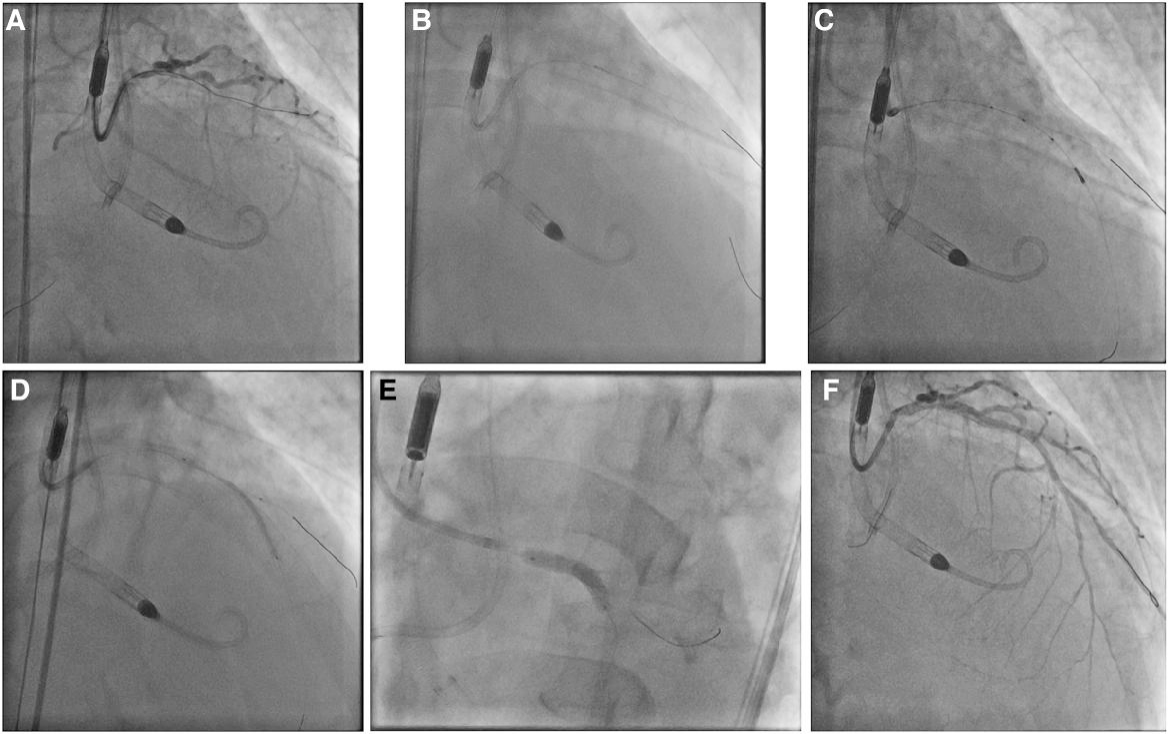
Percutaneous coronary intervention (PCI) of left anterior descending artery (LAD) chronic total occlusion (CTO). (*A*) Gaia Next 1 crossed LAD CTO and passed to 1st diagonal branch. (*B*) Sasuke assisted wiring of distal LAD with Runthrough Floppy wire. (*C*) Intravascular ultrasound (IVUS) interrogation to guide stent implantation. (*D*) 2.25 × 40 mm drug-eluting stent (DES) inserted to proximal and middle LAD. (*E*) 3.5 × 28 mm DES inserted to ostial left main (LM) and proximal LAD. (*F*) Good angiographic results after LM and LAD stenting and post-dilatation.
